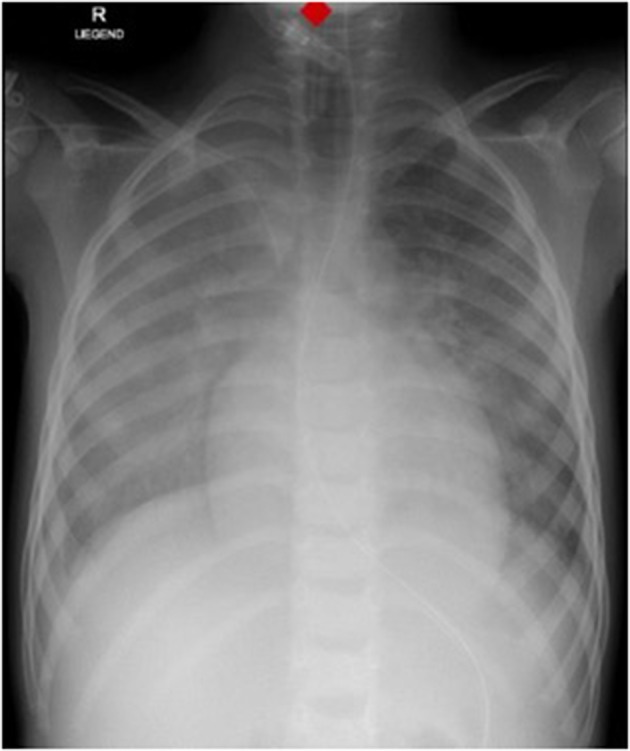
Chest X-Ray showing pulmonary infiltrates and cardiomegaly.
